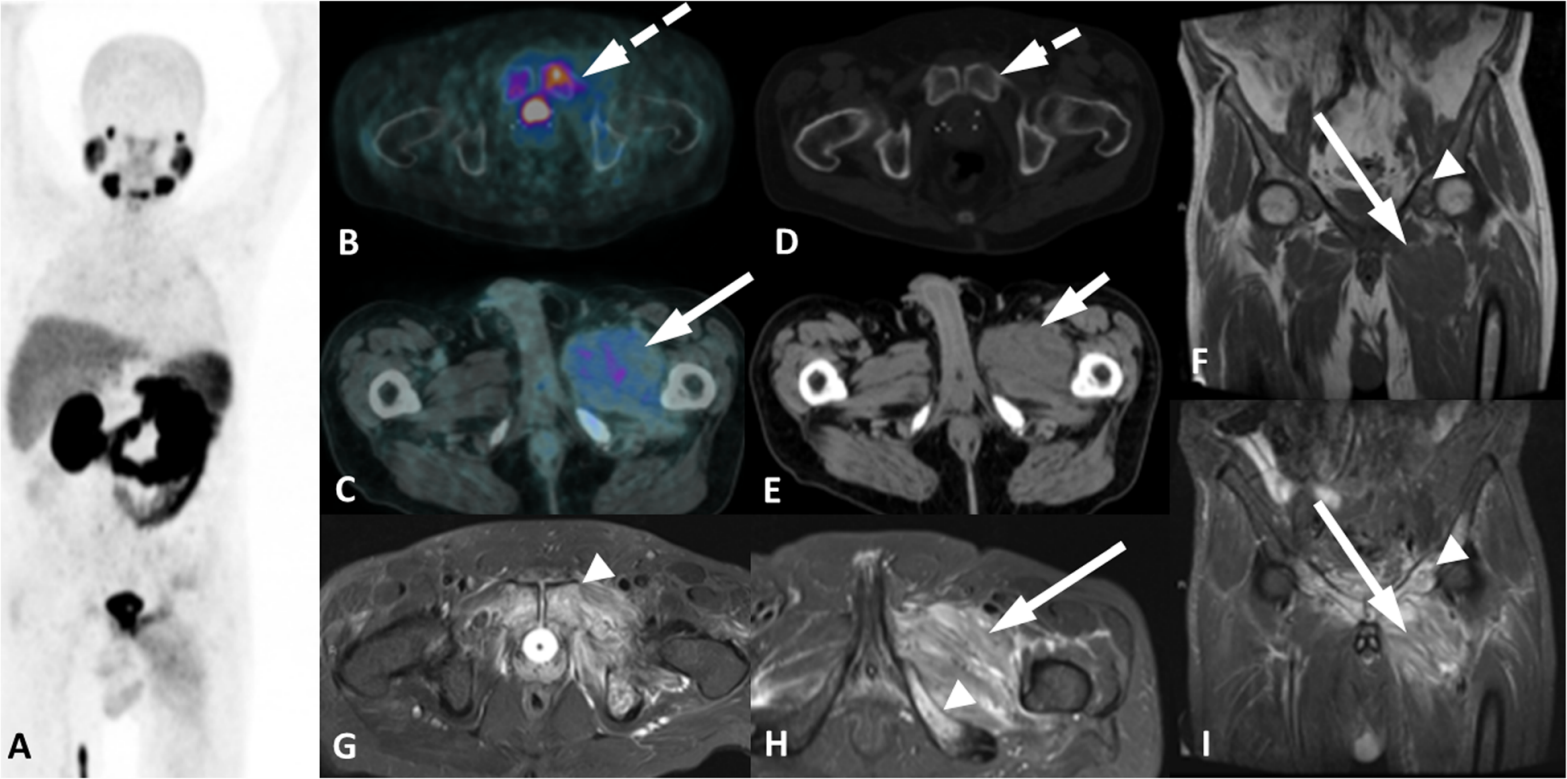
Osteomyelitis with PSMA uptake. ^68^Ga-PSMA-PET/CT MIP (a), axial PET/CT (b, c) and axial CT (d, e) images show a lytic lesion in the left pubic bone with bilateral diffuse bone sclerosis (small dashed arrow) with mild uptake (dashed arrow). Additionally, there is edema in the right adductors muscles (arrow) with discrete and heterogeneous uptake (short arrow). These findings - osteomyelitis (arrowheads) and inflamation in the adjacent soft-tissue (long arrows) - were confirmed on MR images in coronal T1w (f) axial (g, h) and coronal (i) T2w Fat Sat
